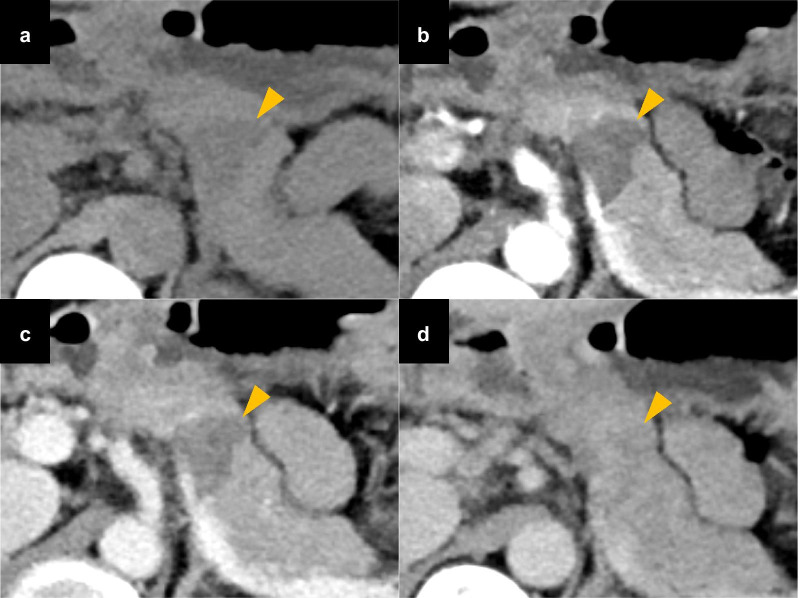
Contrast-enhanced computed tomography showing a gradually enhanced tumor (triangles) at the pancreatic body and tail. a Plain. b Arterial phase. c Portal phase. d Delayed phase
